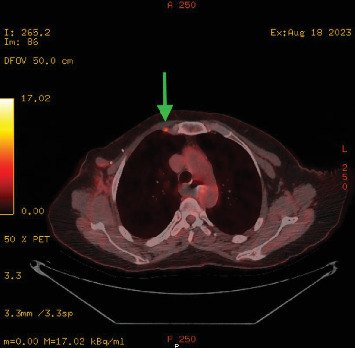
PET-CT, fused axial series, image 86 (18/08/23). Image shows abnormal internal mammary chain node (green arrow).
